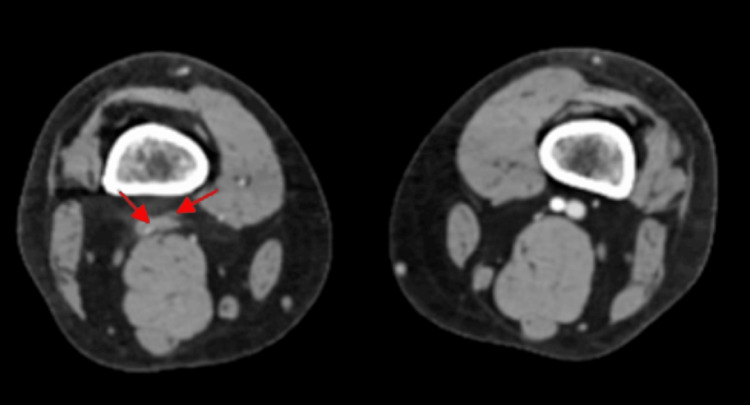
CT angiogram showed occlusion of posterior tibial artery.
